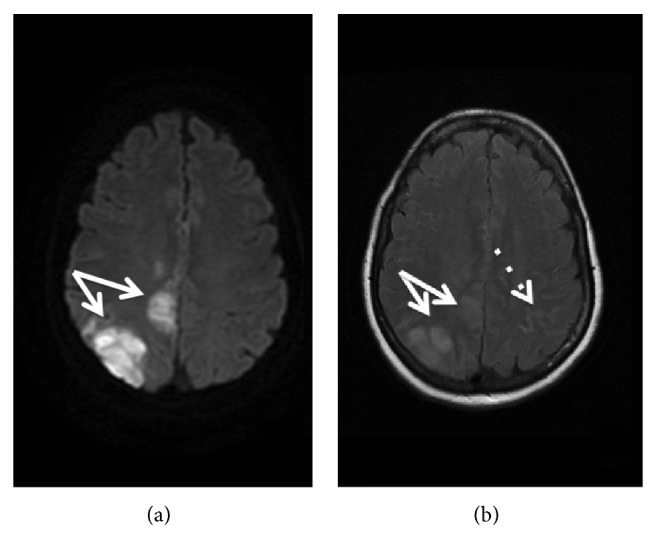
Diffusion-weighted imaging (a) and fluid-attenuated inversion recovery imaging (b) MRI of the patient, showing cytotoxic oedema in the right middle cerebral artery and anterior cerebral artery territories (plain arrows) and cSAH (discontinued arrow).
